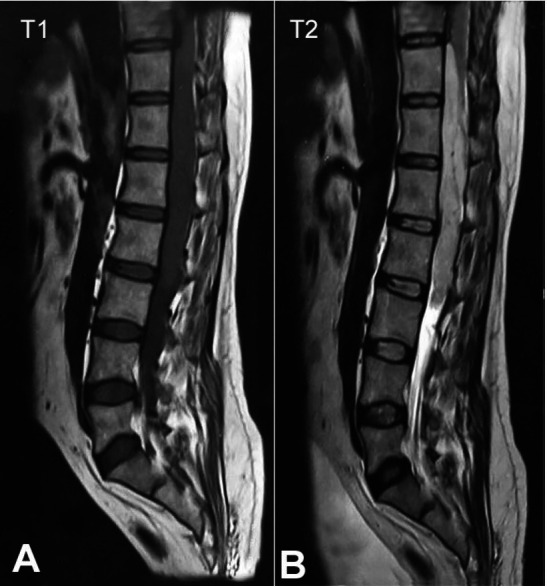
Spinal MRI. A – T1 weighted image – isointense intradural mass measuring 120 × 16 × 25 mm in conus medullaris and filum terminale from D11-L2 without local invasion into surrounding structures; B – T2 weighted image.
